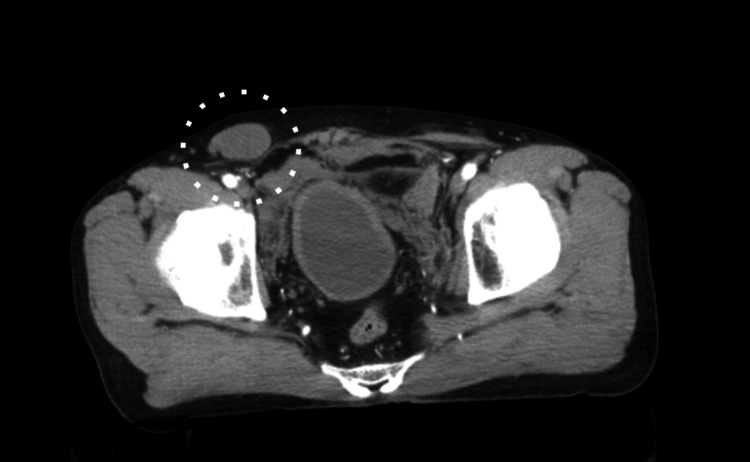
Preoperative axial CT A superficially dislocated right testicle (○) was observed at the level of the acetabulum.
